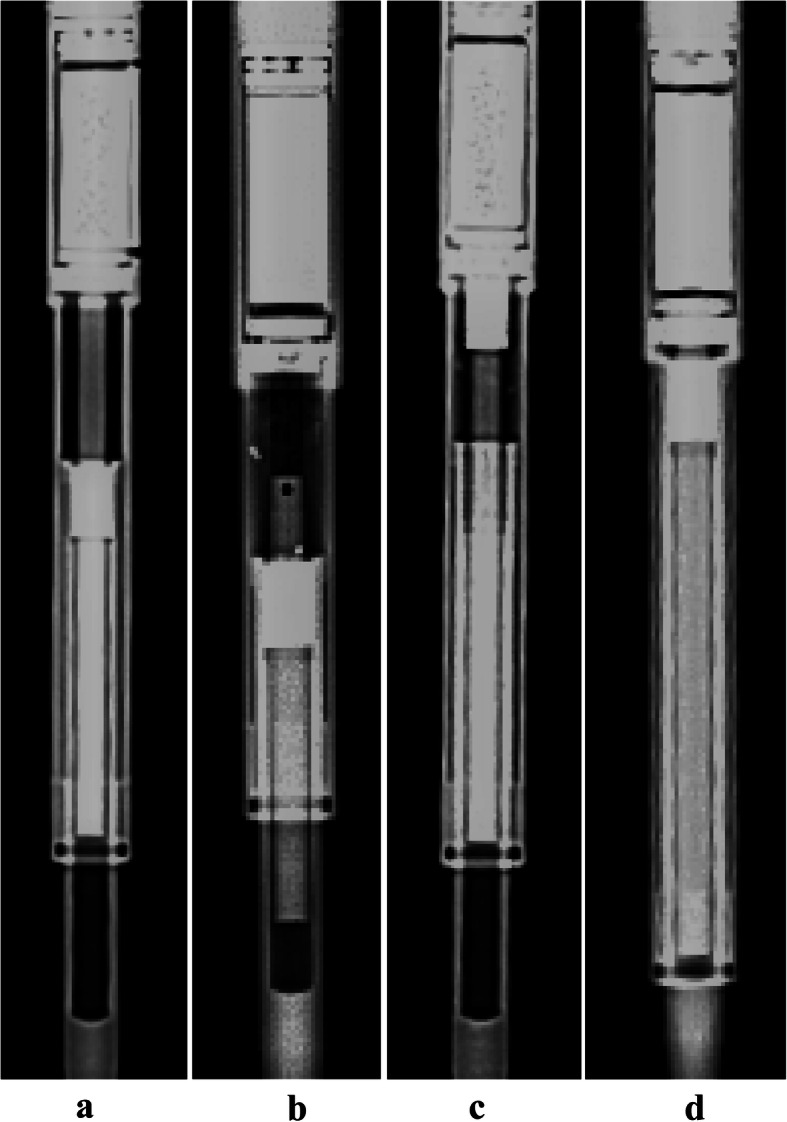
Plain radiographs of four MAGEC rods showing an intact internal mechanism (a), locking pin fracture (b), dislocated internal thread of the telescopic bar (c) and unsure status of internal mechanism (d)
